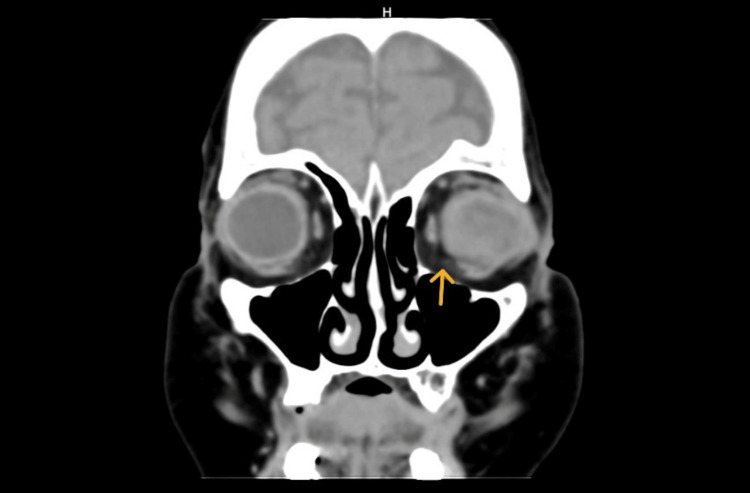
Coronal computed tomography showing rupture of the left globe (orange arrow).
